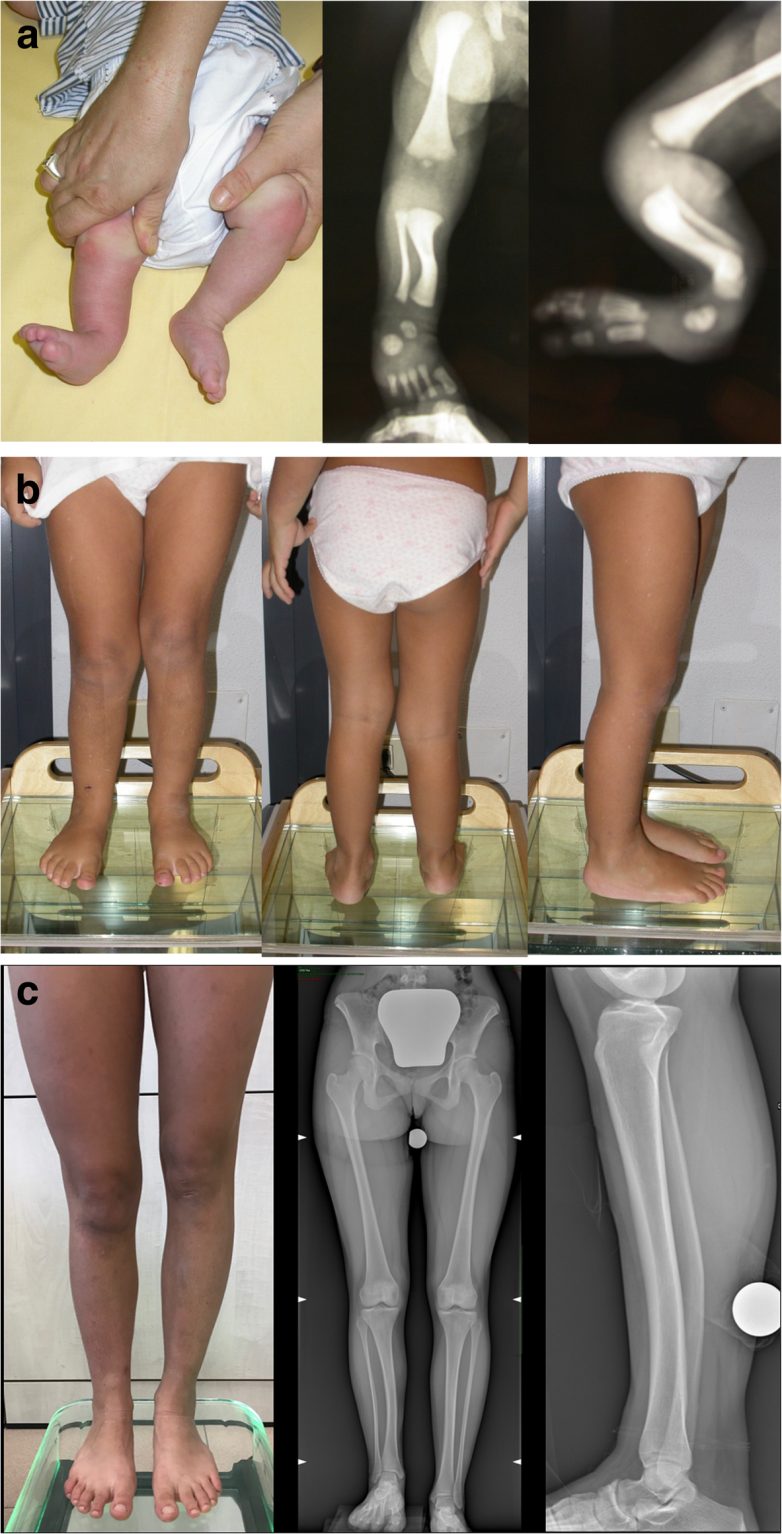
clinical and radiographic appearance of a child presenting with CPMBT, demonstrating the spontaneous progression of the deformity. Figure 3a: at 6 months of age; 3b: at 3.5 years of age; 3c at 15 years of age
